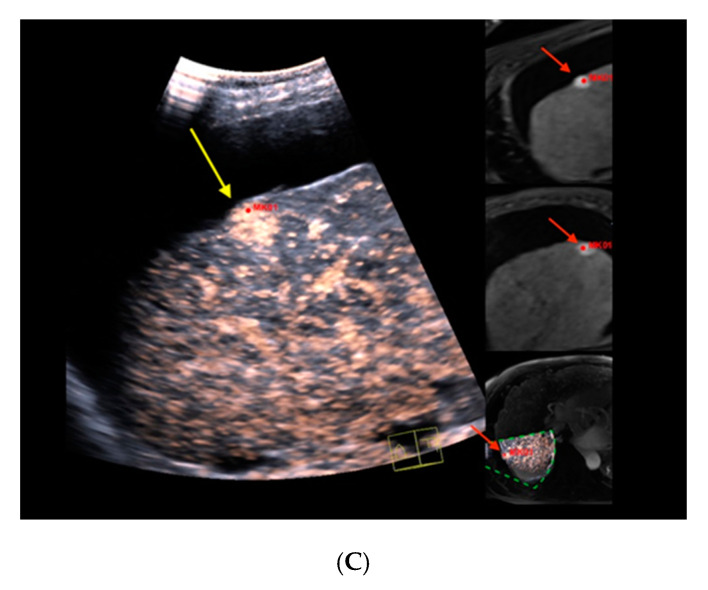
Real-time magnetic resonance imaging-/contrast-enhanced ultrasound (MRI-/CEUS)-fusion imaging of a hypervascularized focal liver lesion in a cirrhotic patient. (A) A hypervascularized subcapsular focal lesion in liver segment 7 was registered in MRI, arterial phase (left), a target lesion (MK01, red) was placed for precise correlation with native B-mode (right) in a side-by-side manner. The focal lesion otherwise was barely detectable by conventional ultrasound. (B) Additional Color Doppler showed livid hypervascularization of the lesion (right), a corresponding plane of MRI, arterial phase (left). (C) Peripheral-to-central contrast-enhancement and, finally, homogeneous contrast enhancement was registered in CEUS (left, maximized), implicating focal nodular hyperplasia. The software interface of the ultrasound device showed four different images: real-time CEUS images (left, maximized), MRI datasets in axial (upper right) and sagittal (middle right) reformation, and a real-time 3D navigation of the MRI-/CEUS-fusion imaging (lower right), (lesion marked by red arrows).
